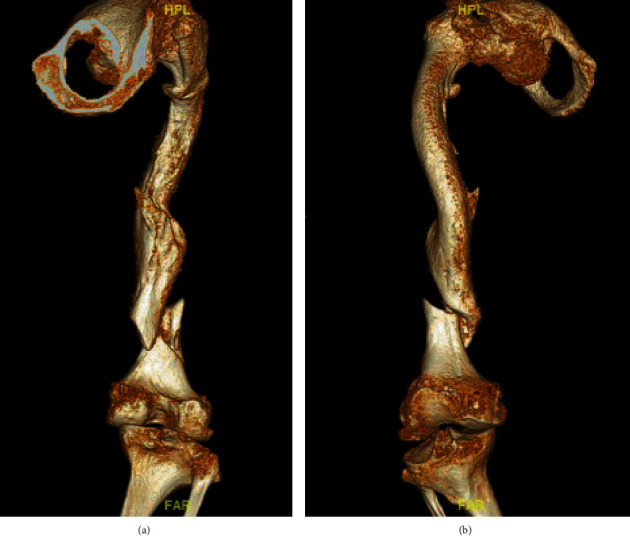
3D computed tomography of the right femur after admission to our emergency department. The images show the fractured femur on the second day after an accident. (a) Femur from dorsal view, where the multifragment characteristic of the fracture can already be assumed. (b) Corresponding ventral view of the femur.
